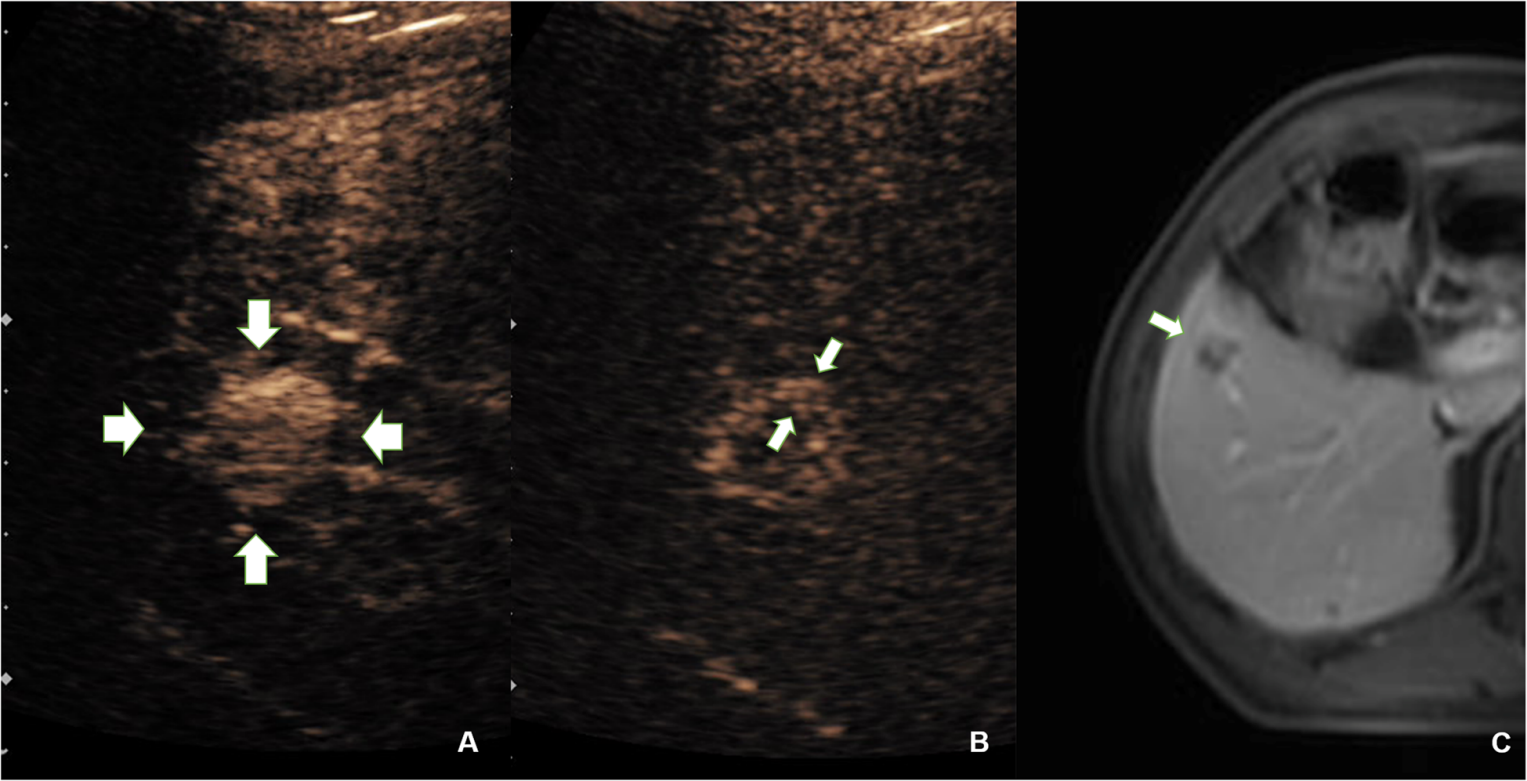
Relationship between presence of corona enhancement in late phase in HA-CEUS and tumour response after TACE. A, Preoperative HA-CEUS revealed a hyperenhanced HCC lesion located in segment 8 (indicated by the arrow). B, Postoperative HA-CEUS showed corona enhancement in the late enhancing phase. C, Postoperative abdominal contrast-enhanced MRI showed a small amount of intratumoural enhancement and peritumoural corona enhancement in portal phase, and the tumour response was defined as partial remission (indicated by the arrow). TACE = transarterial chemoembolization, HCC = hepatocellular carcinoma; CEUS = contrast-enhanced ultrasound; HA-CEUS = hepatic artery contrast-enhanced ultrasound
